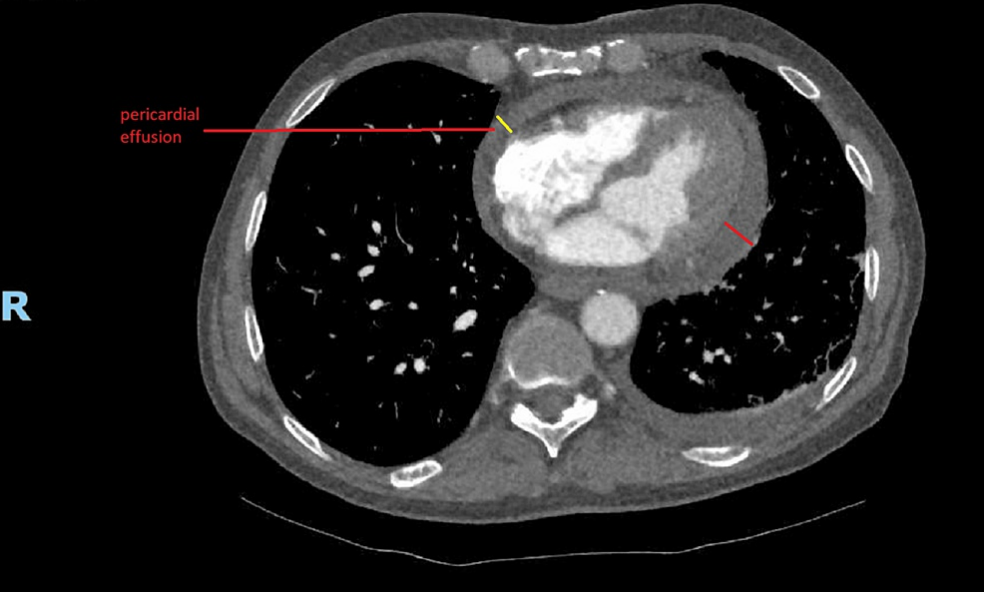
CT pulmonary angiogram CT pulmonary angiogram showing pericardial effusion
